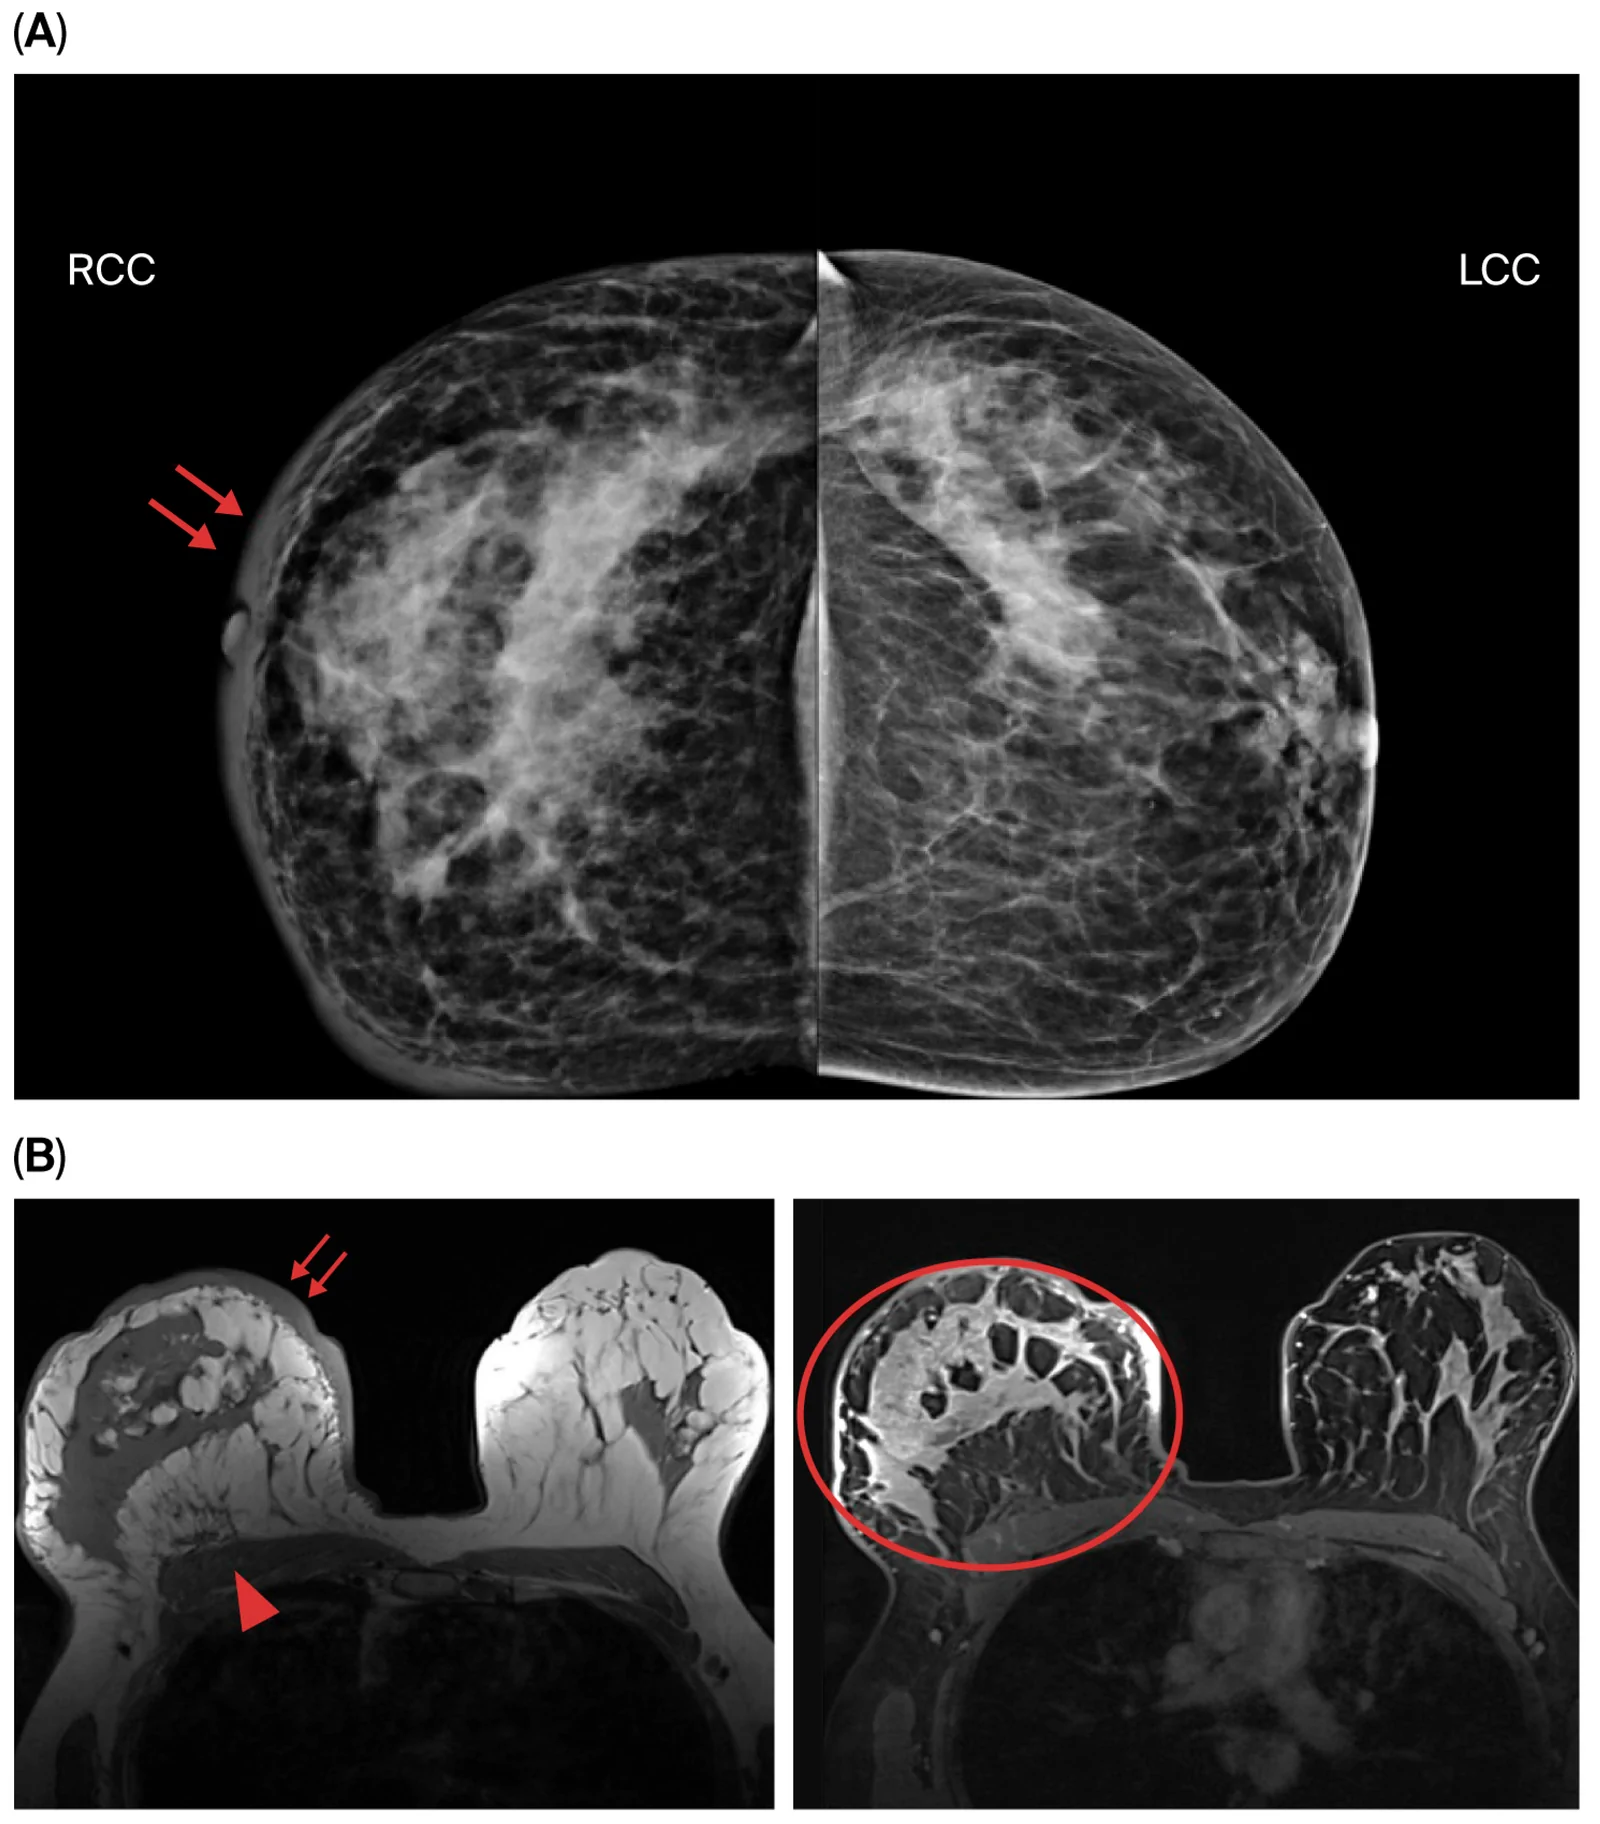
A 34-year-old woman with inflammatory breast cancer. (A) Bilateral CC mammograms. The right CC view demonstrates diffuse skin thickening (arrows) and increased breast density. The left breast appears normal. (B) Axial T2-weighted image and axial contrast-enhanced T1-weighted image demonstrate marked enlargement of the right breast with diffuse skin thickening (arrows), a large isointense infiltrative mass showing diffuse non-mass-like enhancement with a type 3 kinetic curve (circle), and retraction of the right pectoralis muscle (arrowhead). RCC: right craniocaudal, LCC: left craniocaudal.
